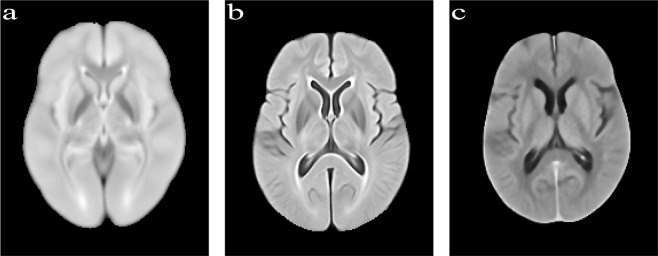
The MIPLAB atlases revealing relatively more detail in boundary regions. (a) Brainder-FLAIR atlas; (b) MIPLAB-FLAIR atlas; (c) MIPLAB-NCCT atlas.
